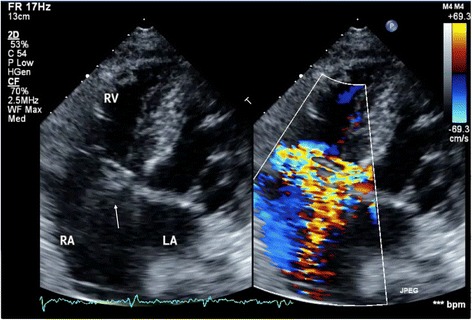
Images of transthoracic echocardiography (TTE) in the apical four-chamber view. *Left* two-dimensional TTE, demonstrating the vegetation attached to the annulus of the aortic valve (*arrow*). *Right* color Doppler TTE, demonstrating the abnormal flow into the right atrium
